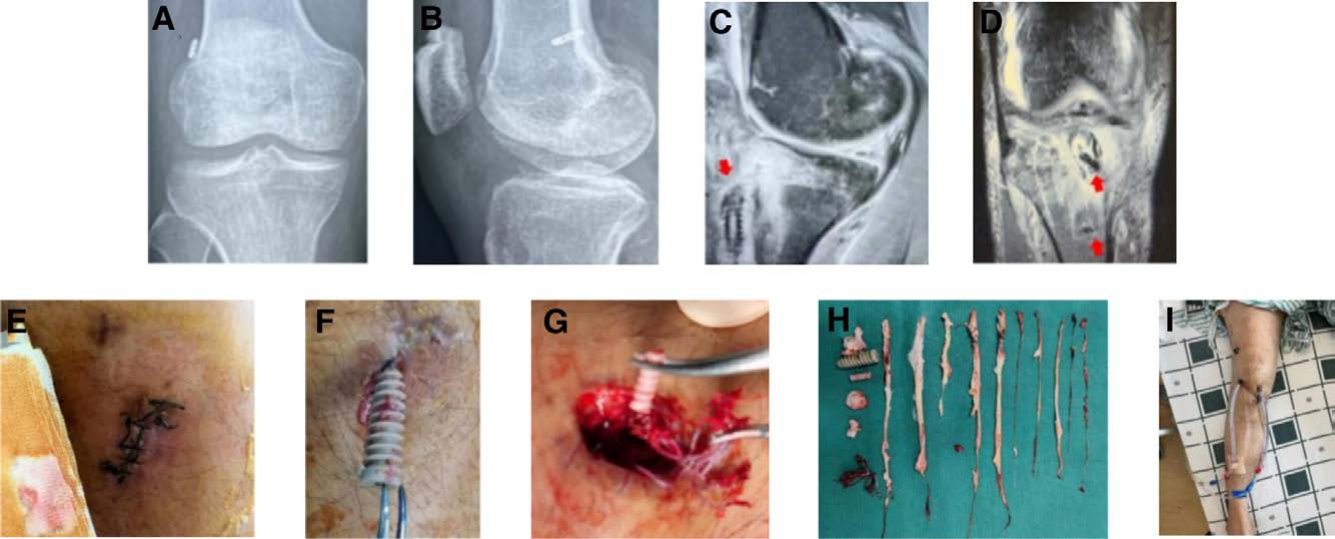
(A and B) DR findings: the femoral and tibial canals were in good positions. (C and D) MRI findings: there was a large amount of inflammation and a notable edema signal in the joint cavity; thus, the tibial bone canal extrusion nail was withdrawn, with an effusion anchor placed below the tibia to fix the suture line of the tendon. (E) The incision where the hamstring tendon of the knee joint was accessed exhibited redness, swelling, and exudation. (F and G) Exudation persisted during the operation at the incision site, necessitating the removal of the tibial extrusion screw and the effusion anchor. (H) The grafted autologous tendon, tibial extrusion screw, and effusion anchor were removed during debridement. (I) The drainage pipe was indwelled for 2 weeks for luminal lavage of the knee joint. When the culture was negative, the lavage was stopped, and the irrigation tube was removed.
